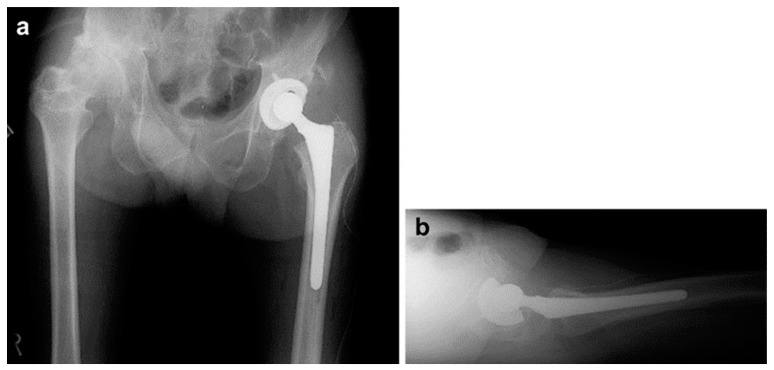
Primary total hip arthroplasty was performed. (a) An anteroposterior plain radiograph of the bilateral hips immediately after primary surgery. (b) Axial image radiograph of the left hip immediately after primary surgery.
